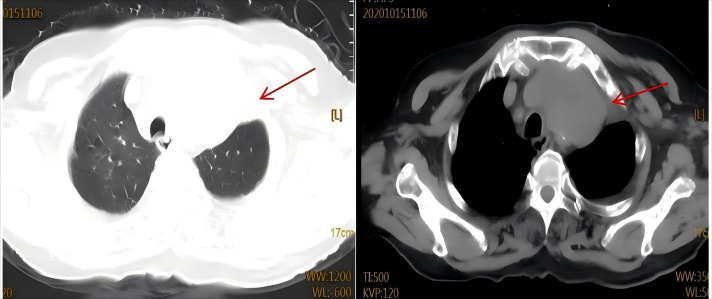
Baseline imaging at initial admission in october 2020.Chest CT scan reveals the anterior mediastinal mass (red arrow).
